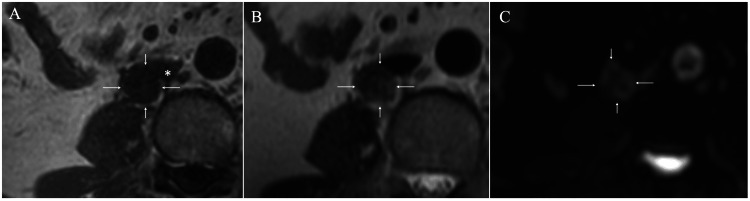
Magnetic resonance imaging (MRI) findings A. MRI of the mass (arrows) showed low signals on T1-weighted images and partially indistinct mass borders adjacent to the inferior vena cava (asterisk). B. MRI of the mass (arrows) showed low signals with faint intra-tumoral high signals on T2-weighted images. C. Diffusion weighted images of the mass (arrows) showed mixed slight high and low signals.
